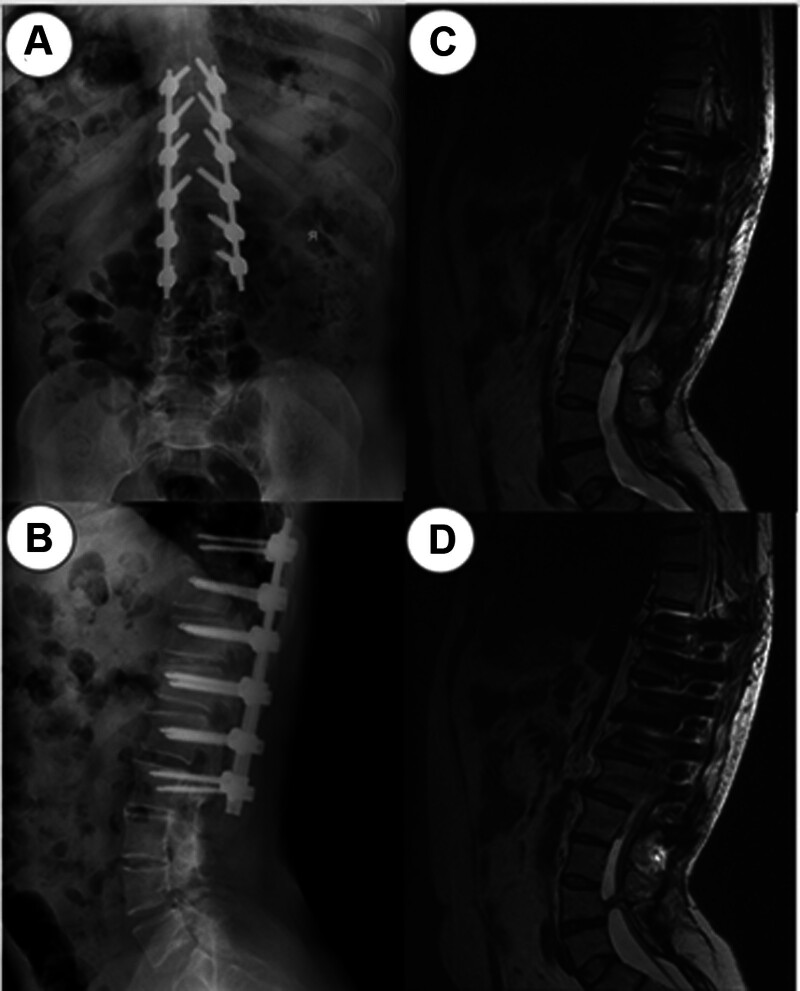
Anteroposterior (A) and lateral (B) plain radiographs of the lumbar spine at 4 months after surgery. Curvature of the spine was well maintained and no re-deformation occurred after correction. (C and D) T2-weighted sagittal MRI of the lumbar spine shows a good internal fixation position. MRI = magnetic resonance imaging.
